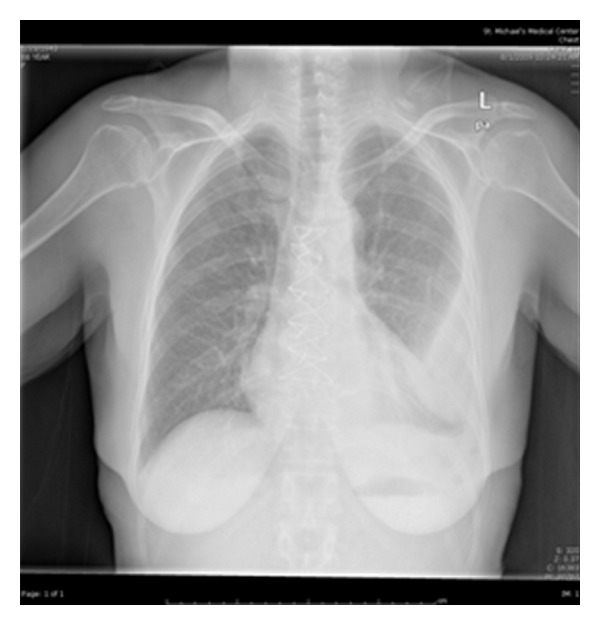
Posteroanterior chest X-ray view of a patient showing opacification of the lower two-thirds of the left hemithorax.
